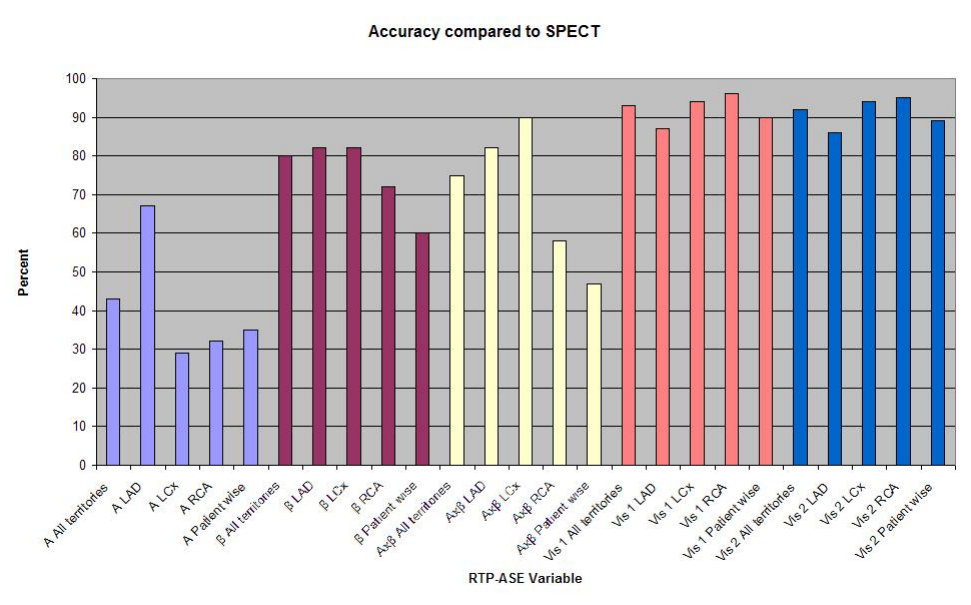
A graph of accuracy between different modalities of RTP-ASE versus SPECT. Left anterior descending (LAD), left circumflex (LCx) and right coronary artery (RCA). Peak signal intensity (A), myocardial blood flow velocity (β), myocardial blood flow (Axβ).
